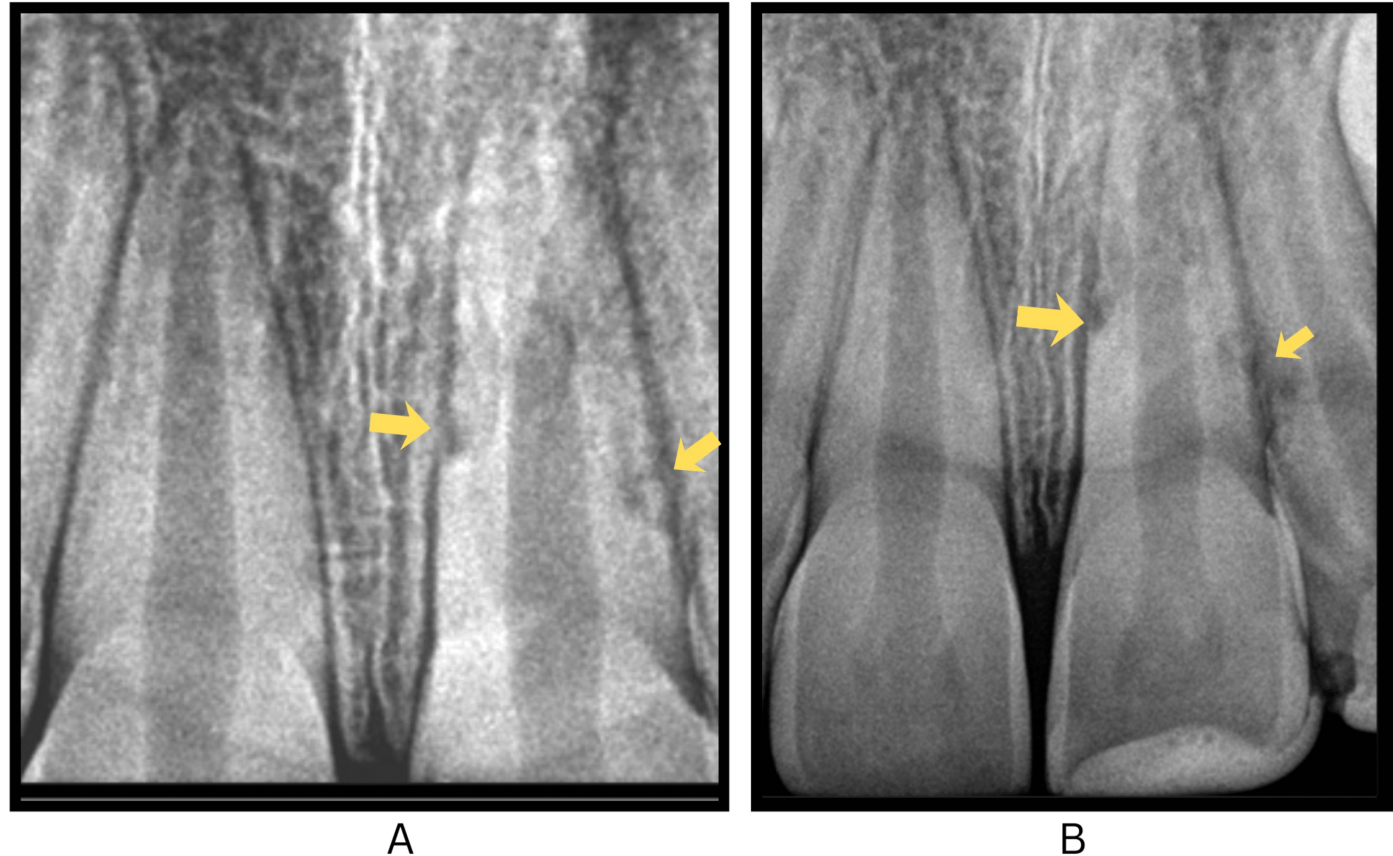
Follow-up periapical radiographs showing localized external root resorption in the replanted maxillary left central incisor. (A) Intraoral periapical radiograph taken four weeks post-replantation showing early changes of resorption marked by arrows on the mesial and distal surface of tooth #21, with the apex remaining open. (B) Intraoral periapical radiograph taken six months post-replantation showing localized resorptive changes on the mesial and distal surface of tooth #21 (marked by arrows), which appear stable and non-progressive. The apex remains immature.
